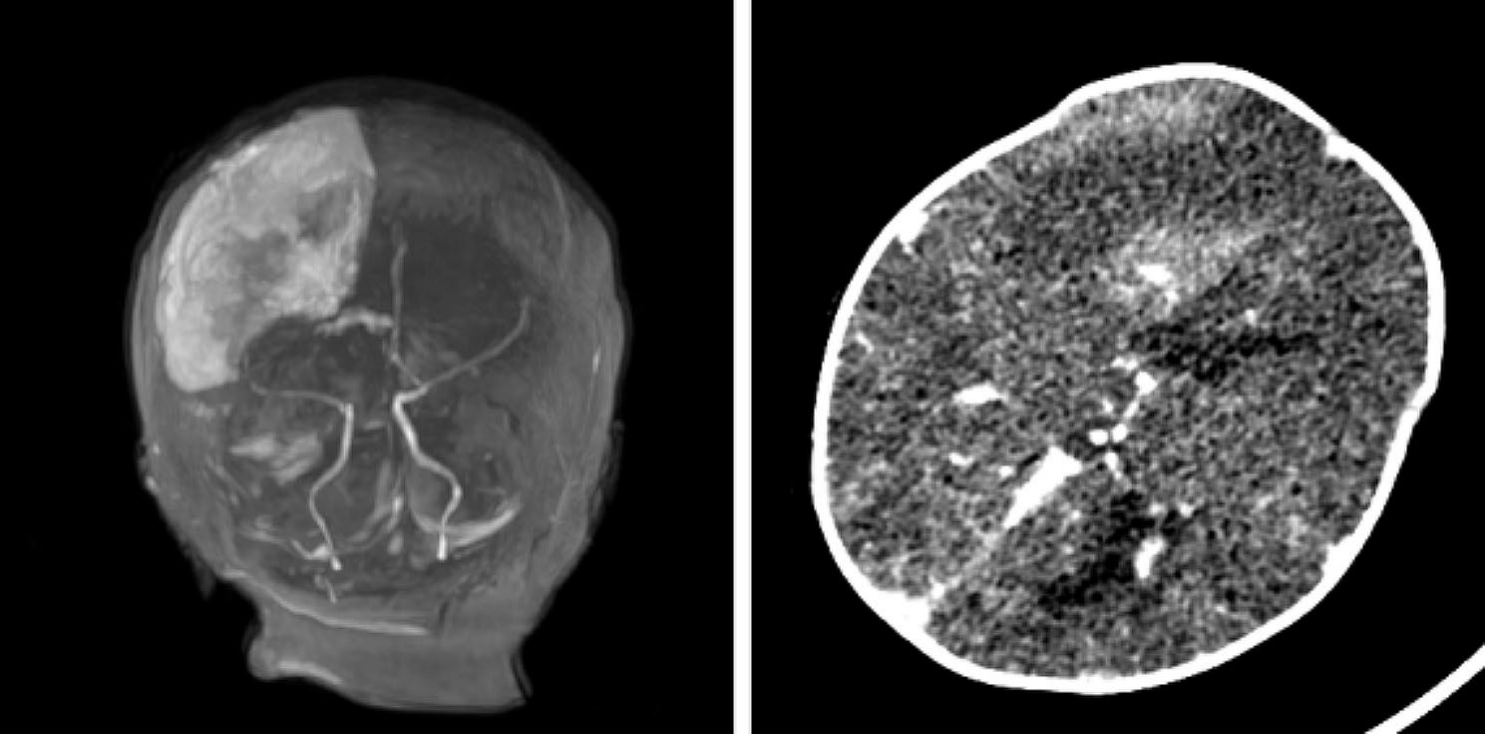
Utility of MRA + MRV and contrast-enhanced CT. TOF MR (Left) angiogram showing poor visualization of the right anterior cerebral artery branches. Contrast-enhanced CT image (Right) showing a vividly enhancing oval mass located directly adjacent to the parenchymal hemorrhage
